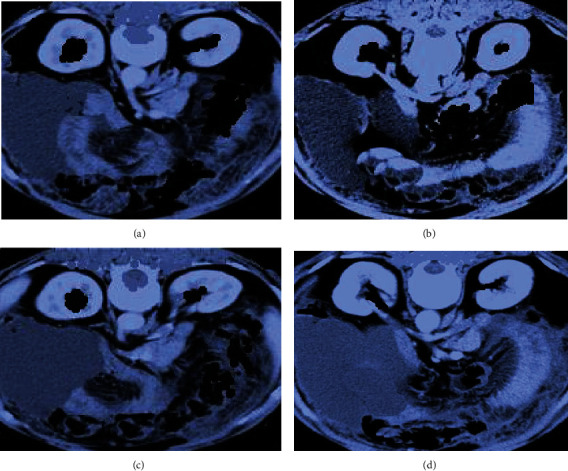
CT image of peritoneal effusion in a 53-year-old male patient with gastric cancer. (a) and (b) were plain CT images; (c) and (d) were enhanced CT images.
